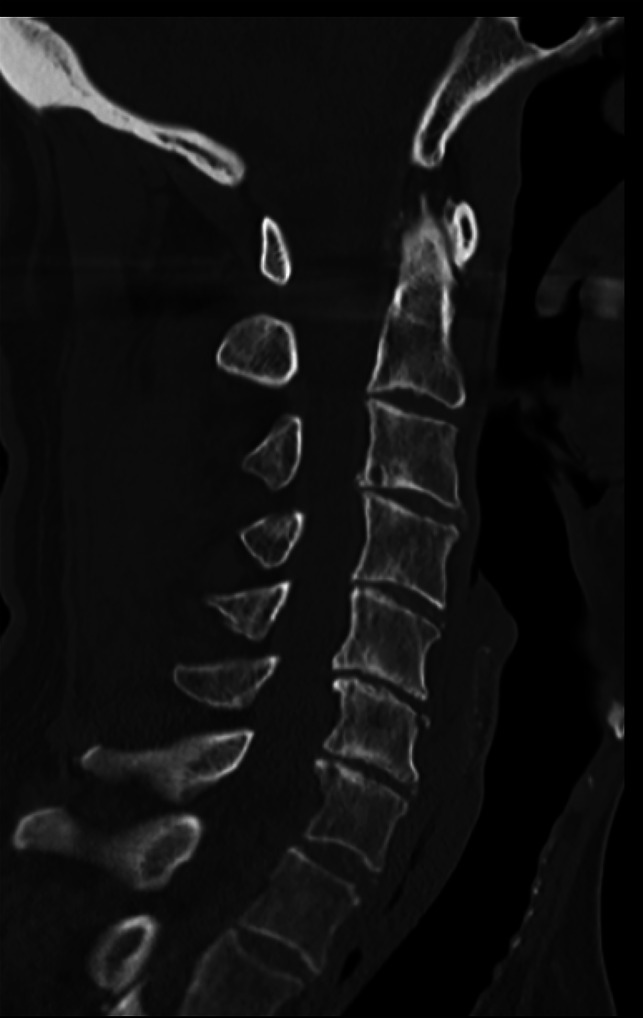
HK CT scan showing degenerative changes but no obvious fracture.
